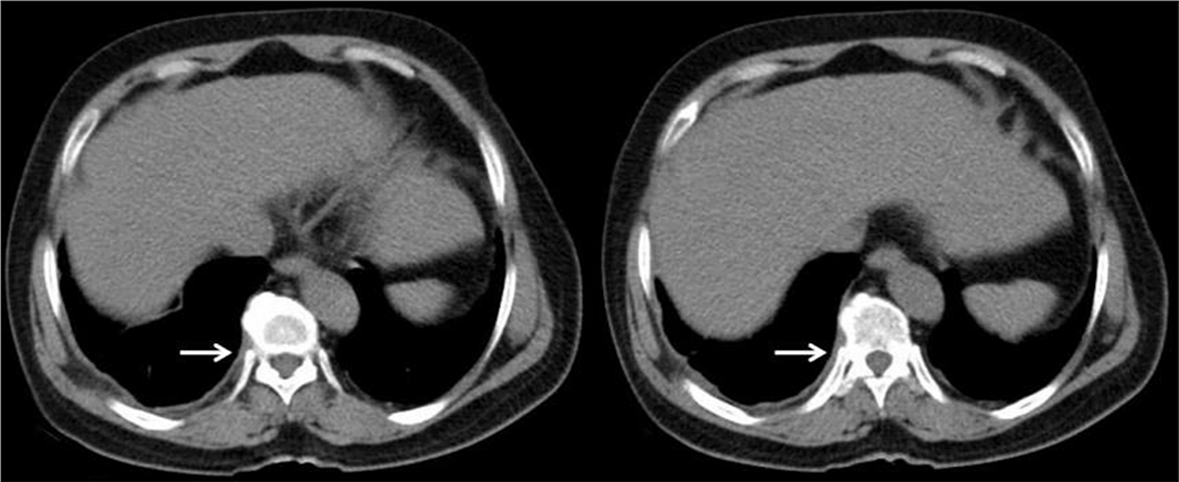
This is a two-slice chest CT image of the patient 4 months after surgery. The white arrow shows that the previous mass is no longer present, no tumor recurrence is seen, and there is pleural thickening after surgery
